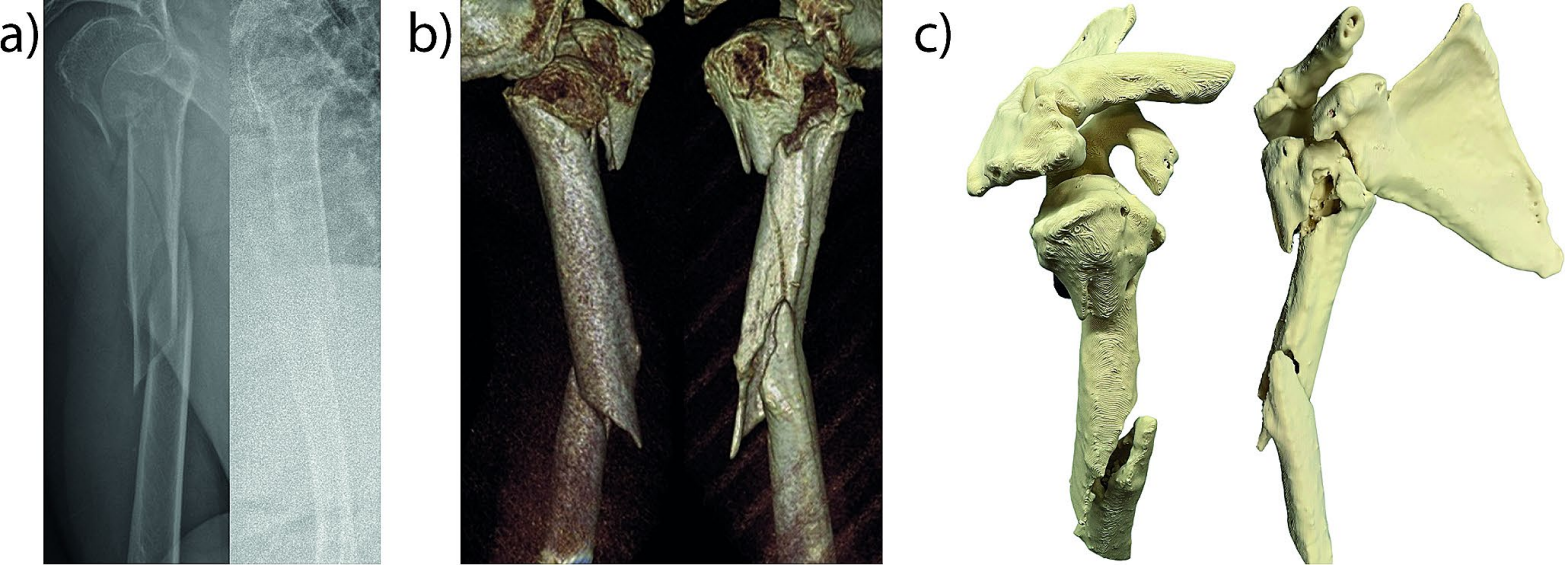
Radiograph, 3D reconstruction, and 3D print of a proximal and diaphyseal humerus fracture (Case 3)
